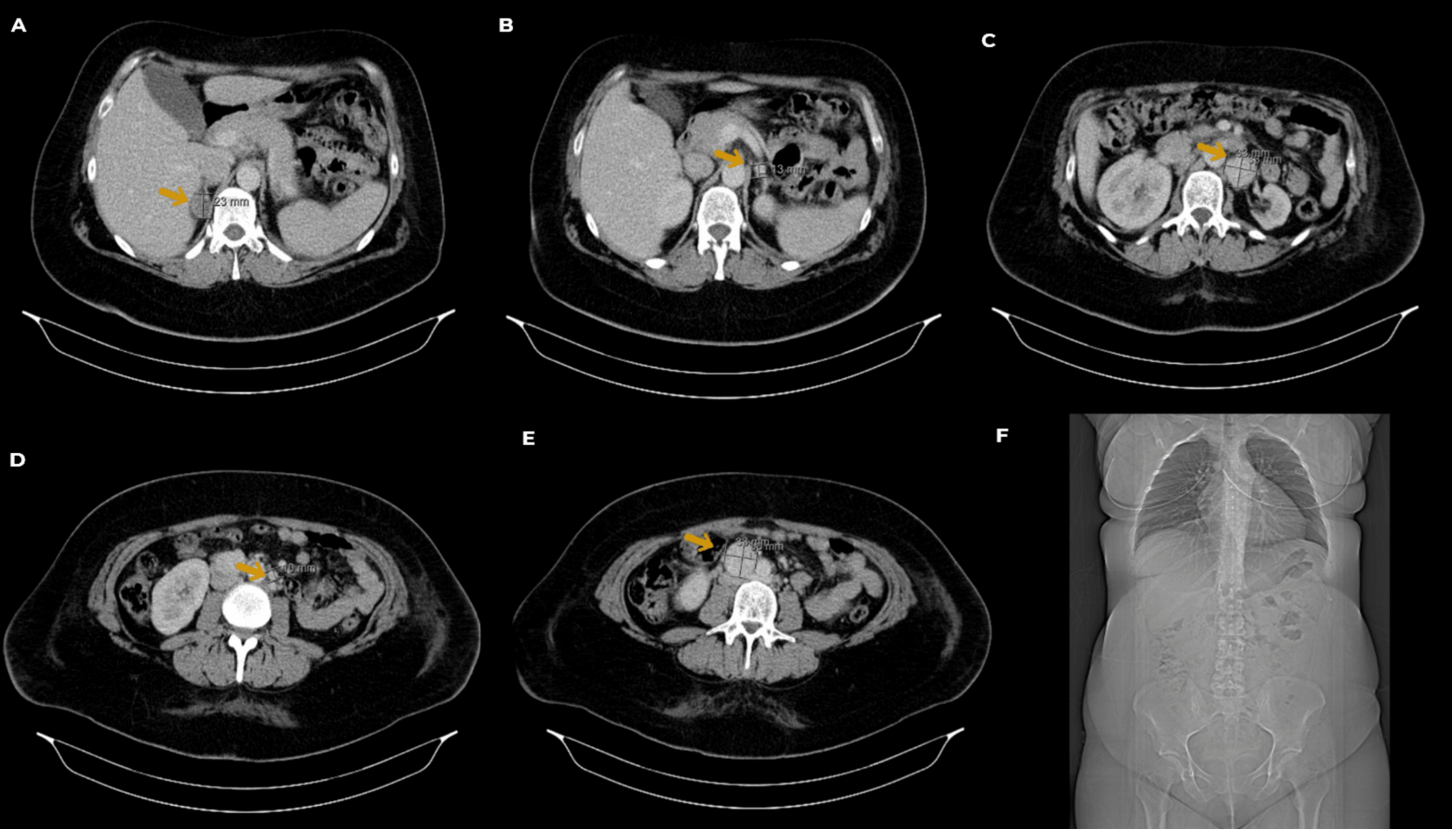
Contrast-enhanced abdominopelvic CT scan performed postpartum The scan revealed the following findings (arrow): (A) a 23-mm mildly enhancing solid nodule in the right adrenal gland, suggestive of an adenoma; (B) a 13-mm hyperenhancing solid nodule adjacent to the left adrenal gland; (C) and (D) two lymph nodes in the left para-aortic region, measuring 33 mm and 10 mm; (E) a 36-mm lymph node in the inter-aortocaval region; and (F) CT scout view providing an overview of the anatomical positioning prior to detailed imaging.
